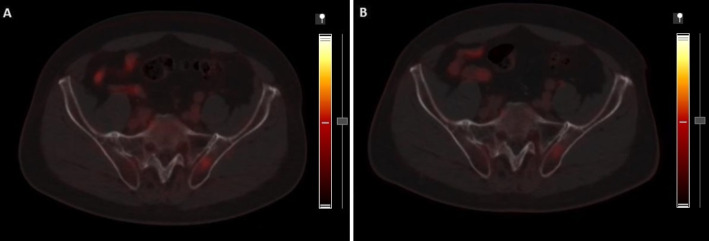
(A) Axial section of PET-PSMA in early phase showing a focal increase in PSMA expression in the left ilium bone (SUVmax 3.7), with no underlying morphological lesion and of doubtful pathological value. (B) Same axial section in second-stage showing decreased PSMA expression (SUVmax 2.4), therefore indicating a nonspecific finding
